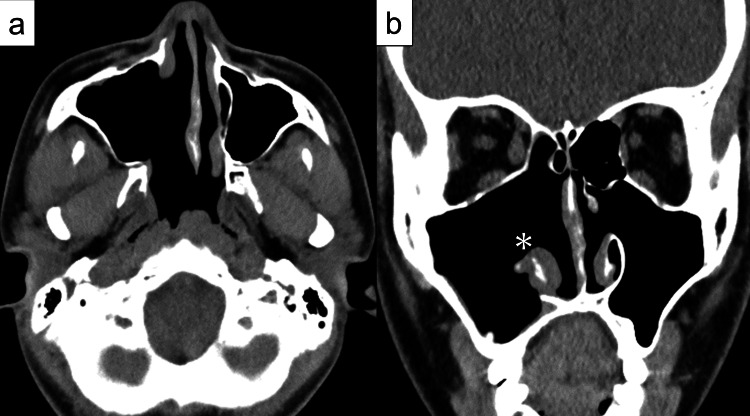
Postoperative computed tomography (CT) findings at 4 months a) Axial image and b) coronal image. CT shows no recurrence of the tumor. *Preserved right inferior turbinate.
